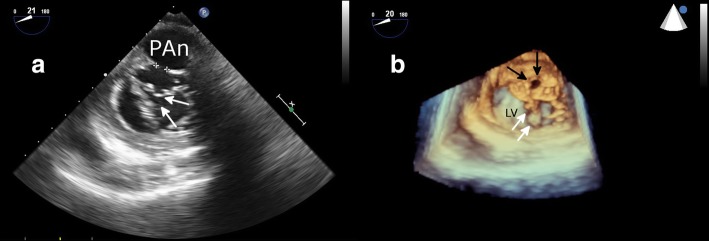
Transesophageal echocardiography. a Two-dimentional image clearly shows the plane struture of the pseudoaneurysm and the neck. b Three-dimensional image shows the neck shape (black arrow). PAn (pseudoaneurysm), LV (left ventricule), White arrow refers to the mitral valve
